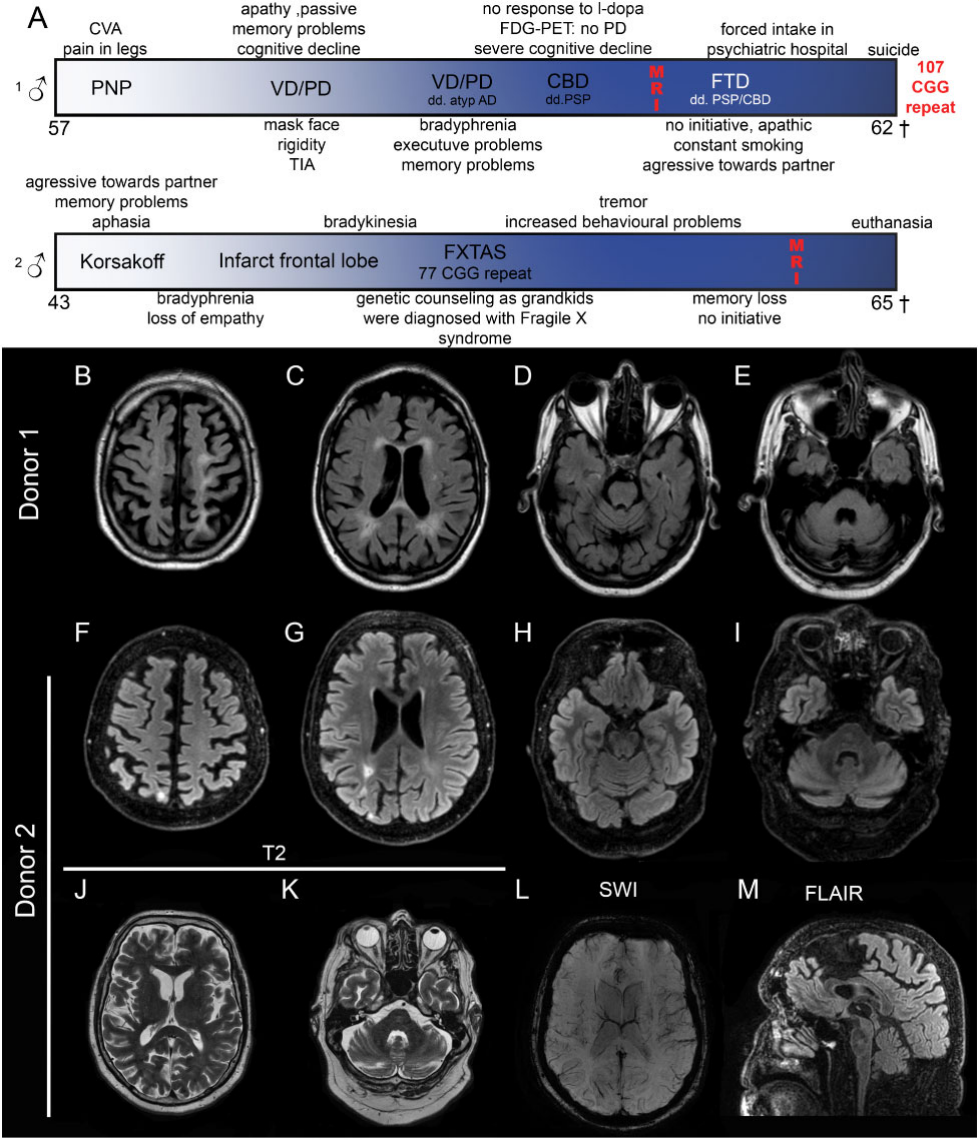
Clinical course and imaging results of Donors 1 and 2. Clinical course (A) shows moment in time of MRI. General mild atrophy is observed (B, F). Ventricles are slightly dilated and Donor 1 shows periventricular white matter lesions (C), Donor 2 shows an increased signal due to an infarct (G). Slight cerebellar atrophy can be seen in both donors (D, E, H, I). Enlargement of the fourth ventricle, but no FXTAS-characteristic middle cerebral peduncle sign can be seen. T2-fluid-attenuated inversion recovery of Donor 2 does not show increased insular and middle cerebral peduncle signal intensities (J, K), no abnormalities in vasculature (K) and no thinning of the corpus callosum (M). CVA, cerebrovascular accident; PNP, polyneuropathy; VD, vascular dementia; PD, Parkinson’s disease; AD, Alzheimer’s disease; CBD, corticobasal degeneration; FTD, frontotemporal dementia; PSP, progressive supranuclear palsy.
